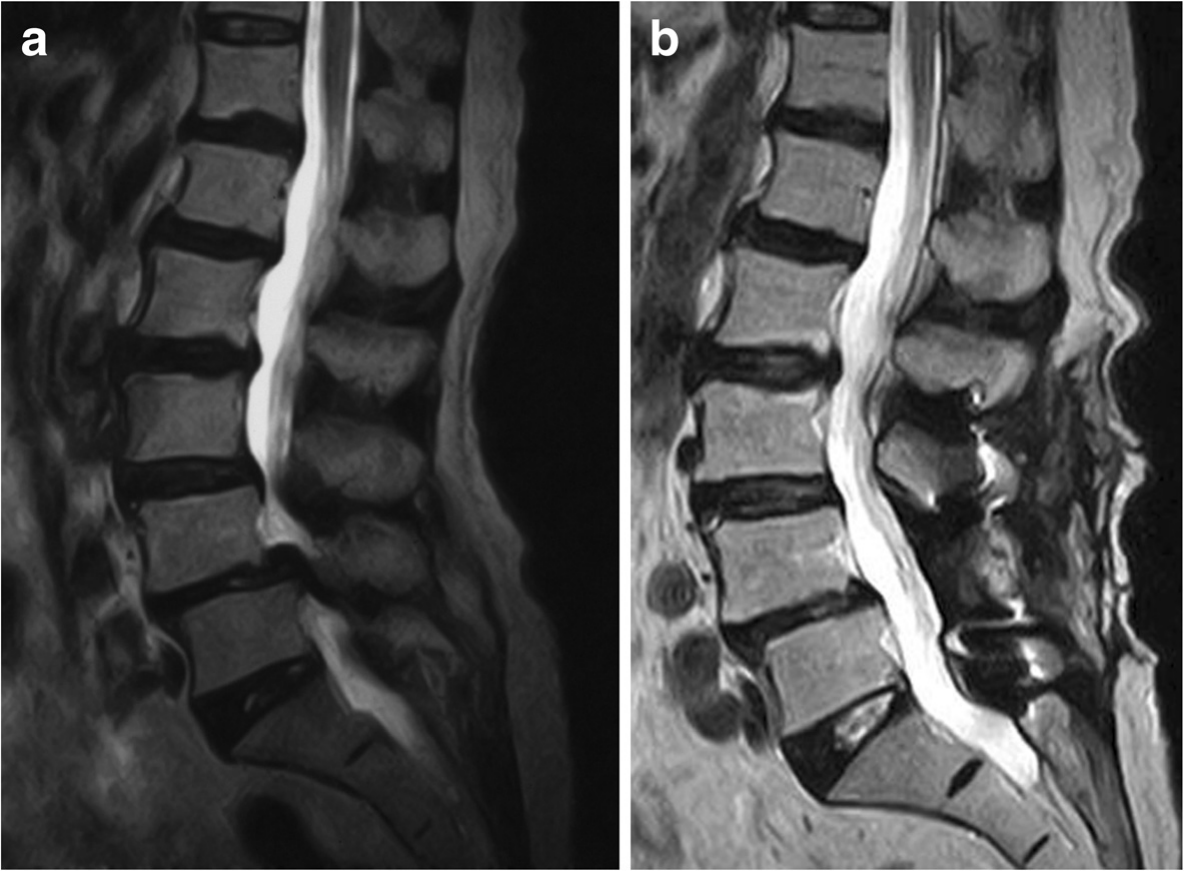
MRI of a 76-year-old female with spondylolisthesis. (a) Preoperative T2-weighted image. (b) Postoperative T2-weighted image.
